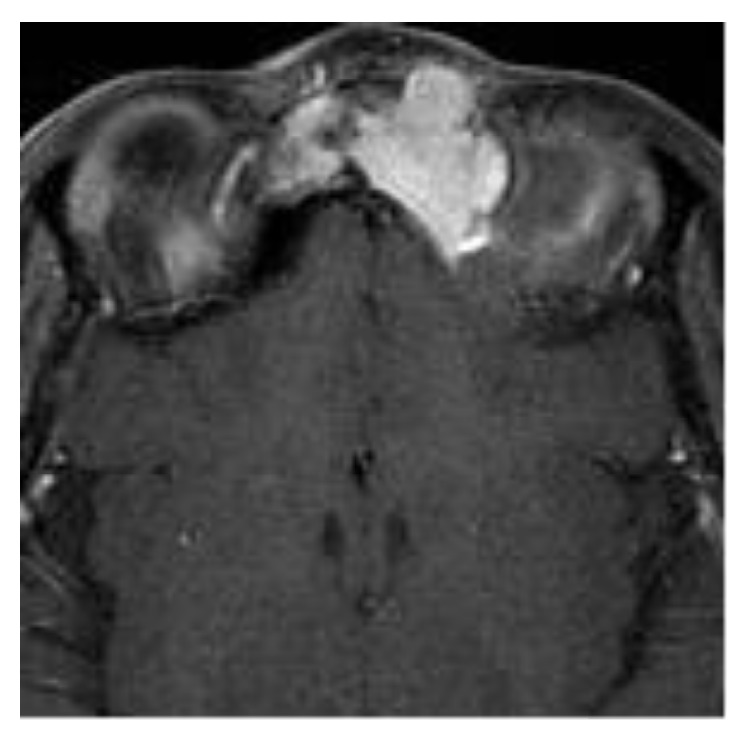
Preoperative axial MRI image of the tumor.
